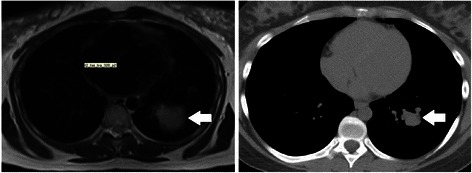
Chest MRI and CT examination before (left) and after (right) surgical abortion. The volume of the largest pulmonary manifestation decreased from 30.02 cm^3^ to 3.51 cm^3^
